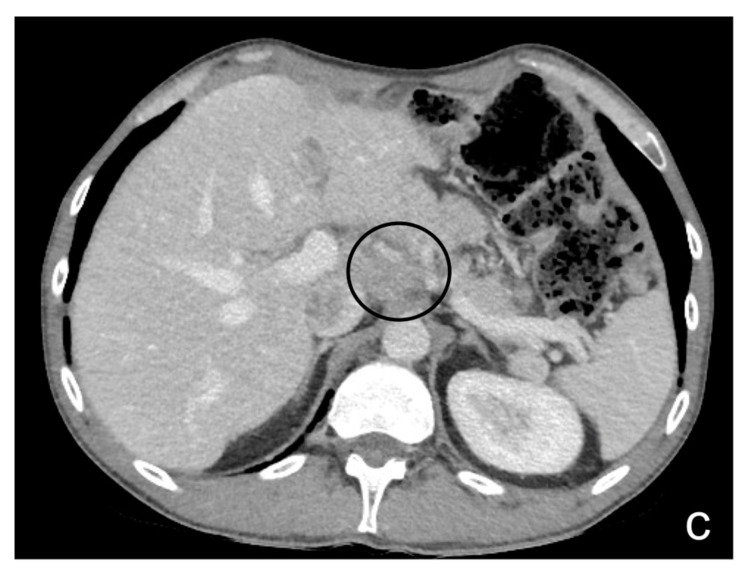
Contrast-enhanced CT scan, axial views. Case of an infiltrating-type gastric adenocarcinoma in a male, 40 y. Nodule of peritoneal carcinosis (black circle), localized on the Glissonian surface (a) in the fold of the falciform ligament (b) in the perihilar area (c).
